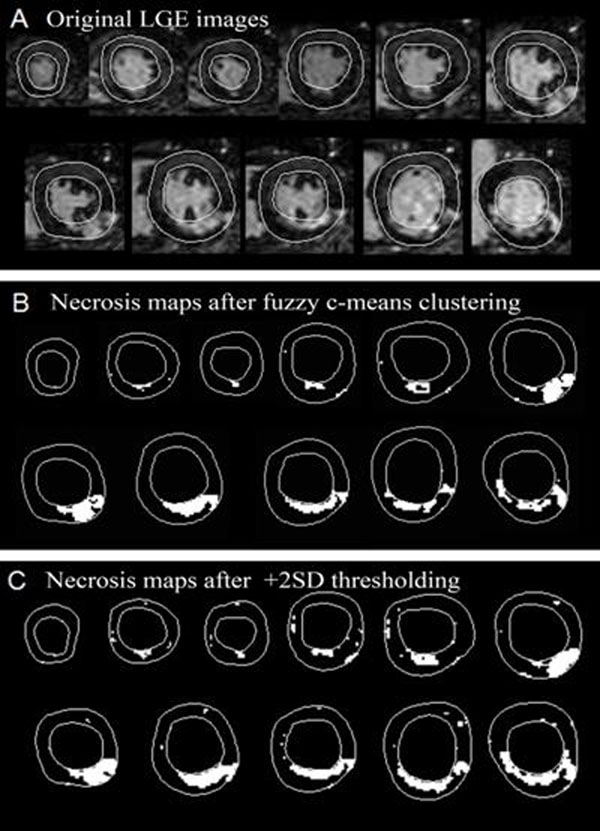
# Fuzzy-logic, manual and semi-automated 2SD-based approaches for quantification of myocardial necrosis from late contrast enhancement magnetic resonance images: comparison with biochemical assessment of infarct size and left ventricular volumes and function early after myocardial infarction
